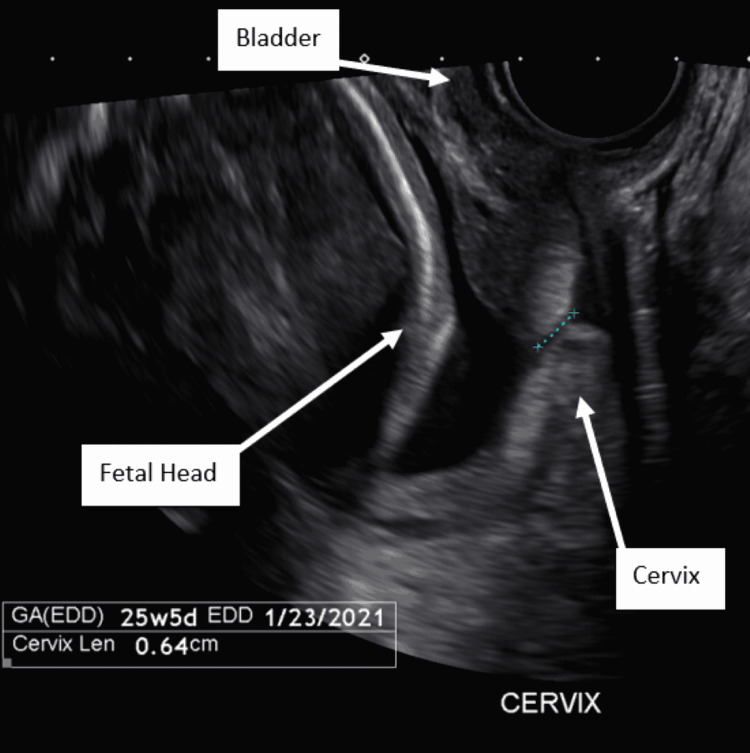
Transvaginal ultrasound cervical length at the time of presentation (0.64 cm).
